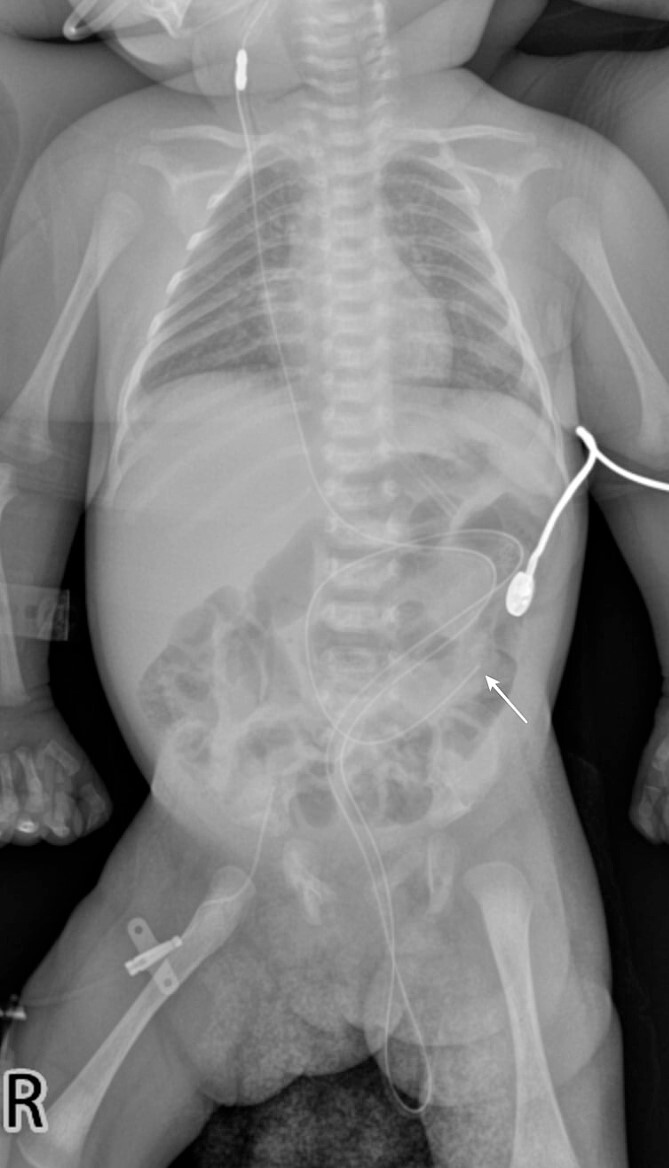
A babygram X-ray demonstrates the distal tip of the shunt positioned at the level of the left paravertebral L4 (white arrow), with a portion of the shunt extending downward and protruding from the abdominal cavity.
